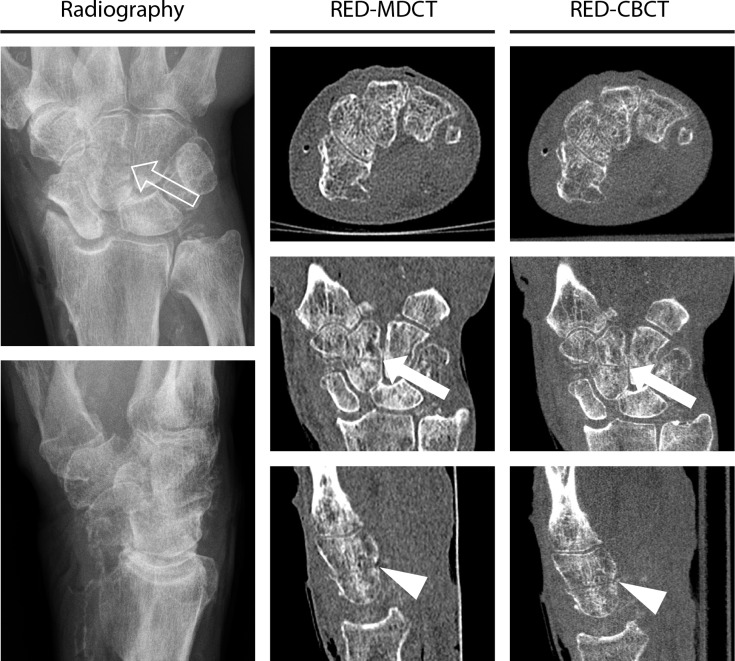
Imaging examples of one case for radiography (d.p./ lat.), radiography equivalent dose multidetector CT (RED-MDCT) and radiography equivalent dose cone-beam CT (RED-CBCT) with axial, coronal and sagittal reconstructions. The fracture of the capitate is clearly shown in the CT images (white arrows and white arrowheads), whereas radiography depicts the fracture only faintly (arrow).
